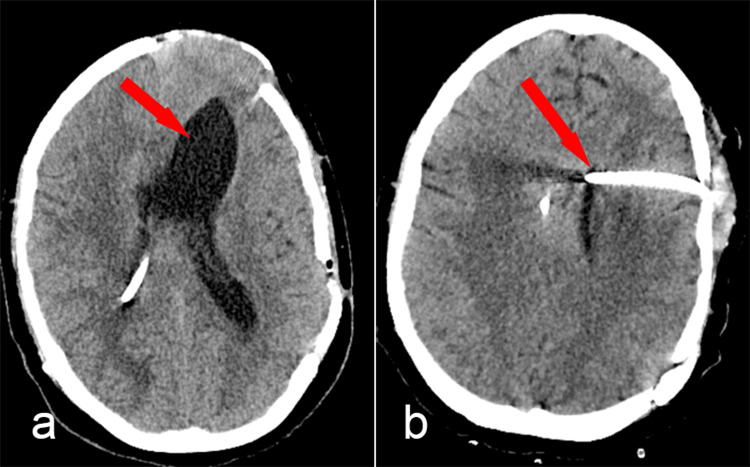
a: CT scan showing functional right ventricle catheter and dilated left ventricle (red arrow); b: CT scan after the insertion of the second catheter into the left ventricle (red arrow)
